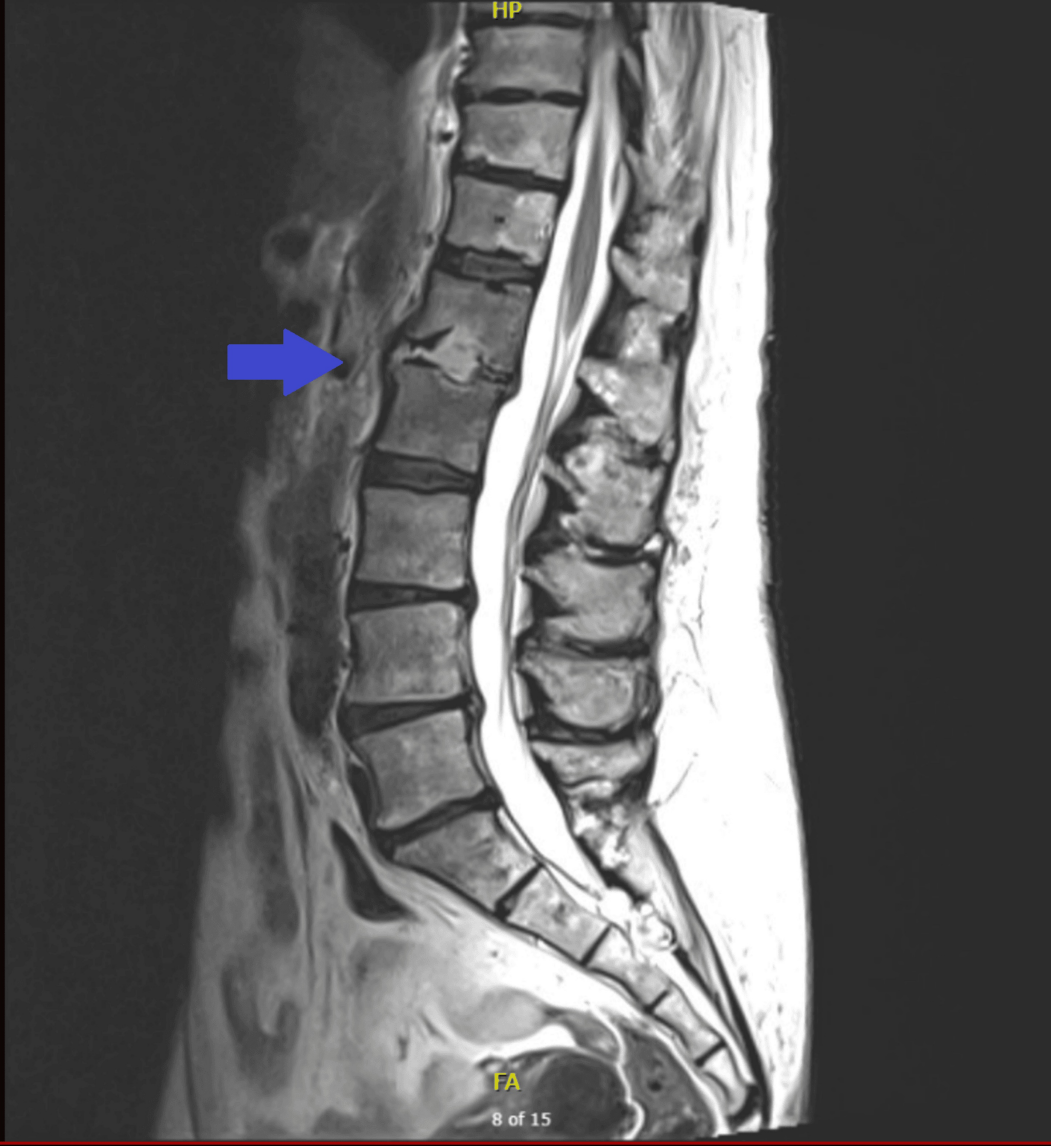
MRI lumber spine revealing abnormal high signal within the L1/L2 disc, erosions, and bone marrow oedema of the opposing vertebral endplates.
